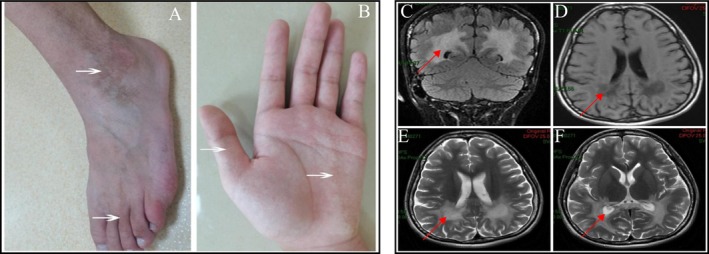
Analysis of skin pigmentation and MRI characteristics in the X‐ALD proband. (A, B) The proband exhibits marked pigmentation in specific areas of the skin. As indicated by the white arrows in the images, significant pigment deposition is observed in the ankles, toes, and palms. (C–F) MRI scans of the proband's brain reveal lesions primarily localized to the white matter surrounding the posterior region (C), posterior horns (D, E), and inferior horns (F) of the bilateral lateral ventricles, with involvement of the corpus callosum. On T1‐weighted imaging (T1WI), these lesions appear as areas of hypointensity, whereas on T2‐weighted imaging (T2WI), they demonstrate hyperintensity. The region denoted by the red arrow demonstrates an abnormal signal within the white matter.
